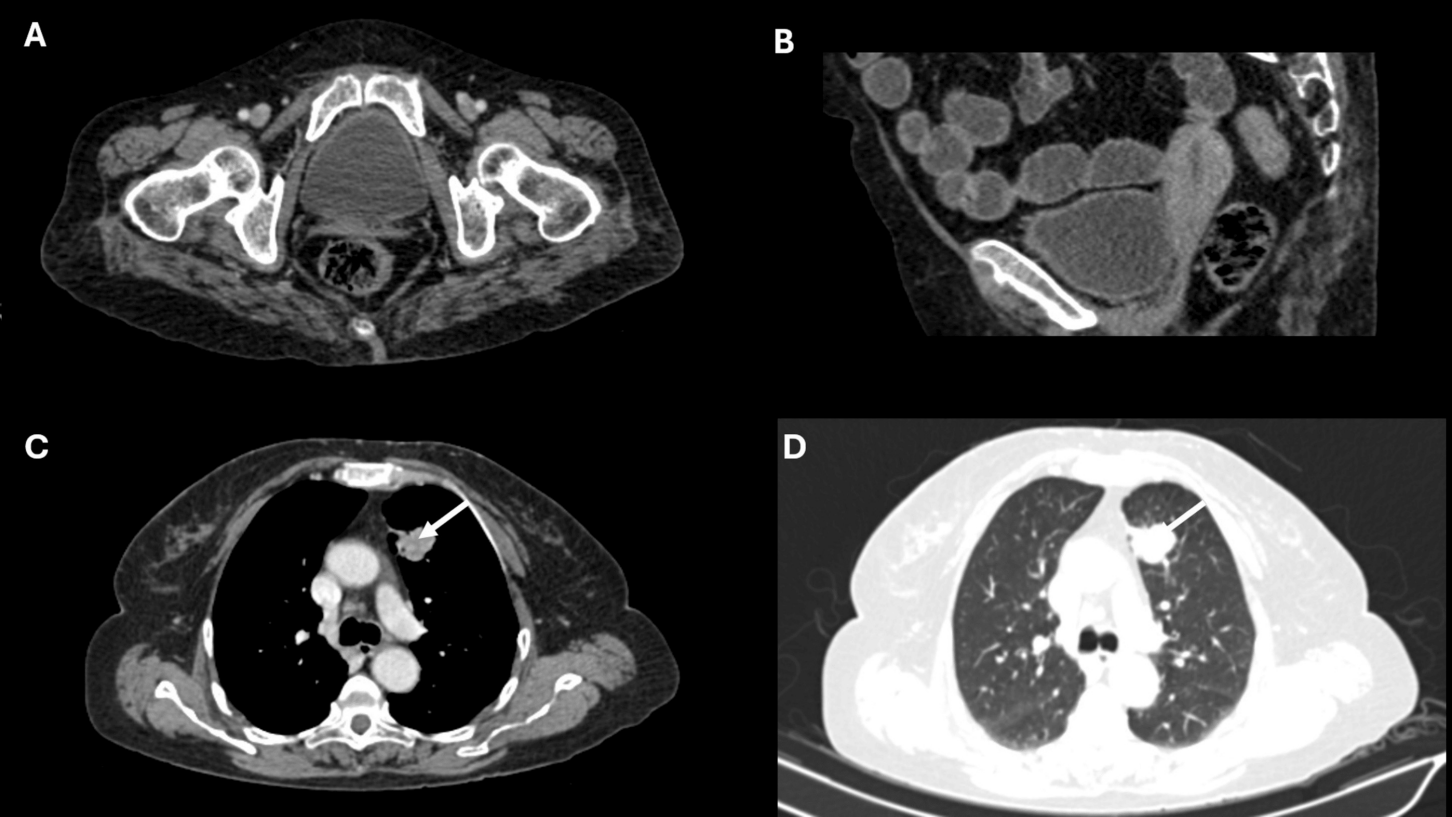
Cross-sectional (A) and sagittal (B) CT images reveal no evidence of enhancing lesions involving the cervix. Cross-sectional images (C & D) reveal fibroreticular opacities in the region of the previously seen lesion in the anterior segment of the left lung upper lobe (white arrows).
